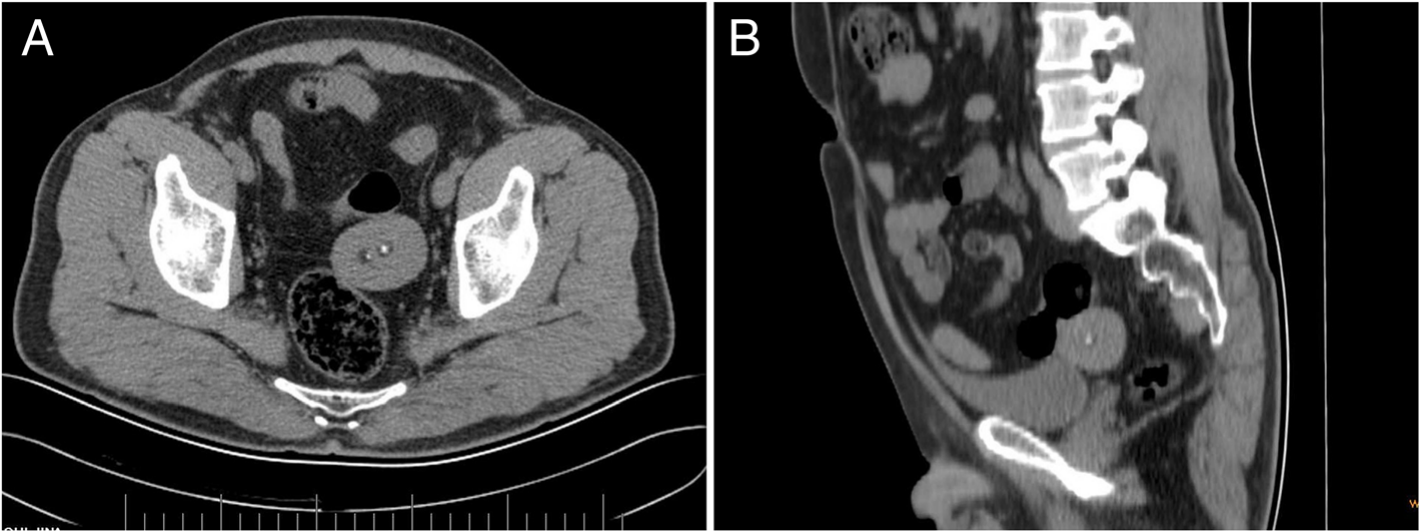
Abdominal computed tomography findings. (A) Axial image demonstrated a low-density lesion with complete capsule and two calcifications in the central part. (B) Sagittal image showed the mass adjacent to the sigmoid colon in the left iliac fossa.
